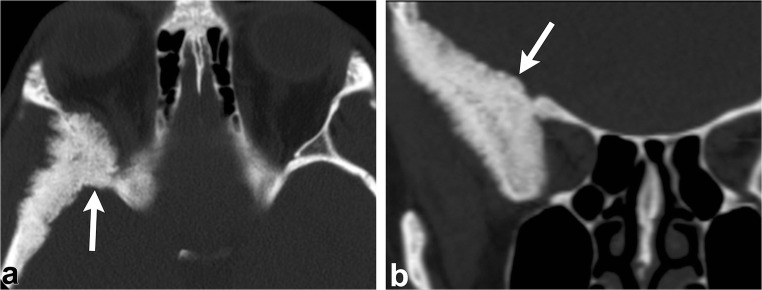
Intraosseous meningioma. Axial (a) and coronal (b) CT images portray an intraosseous meningioma in the right sphenoid extending into the right lateral orbital wall (*arrows*). Note the expansion of bone and “prickly” border of the lesion
